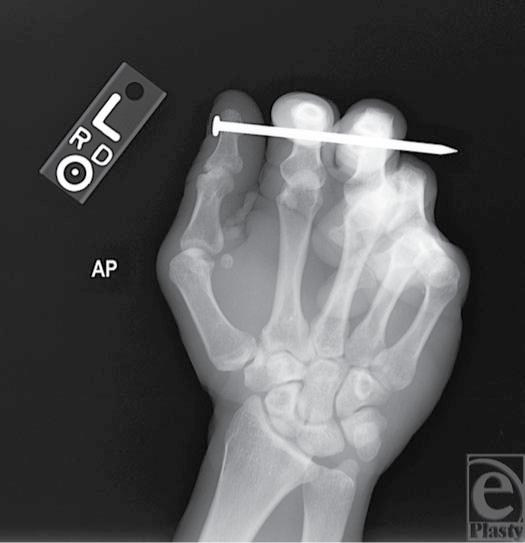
X-ray film demonstrates no bony injury to phalanges (AP [anteroposterior] view).
